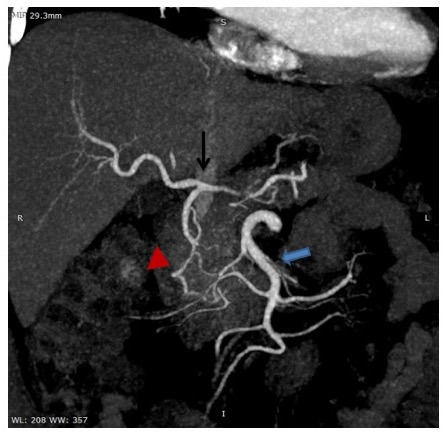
CT angiography image on which the main branches are marked. Superior Mesenteric Artery (thick blue arrow), Common Hepatic Artery (thin black arrow), Arc of Buhler (red arrow head). Although the celiac trunk is almost occluded, blood flow to organs is preserved by the Arc of Buhler.
